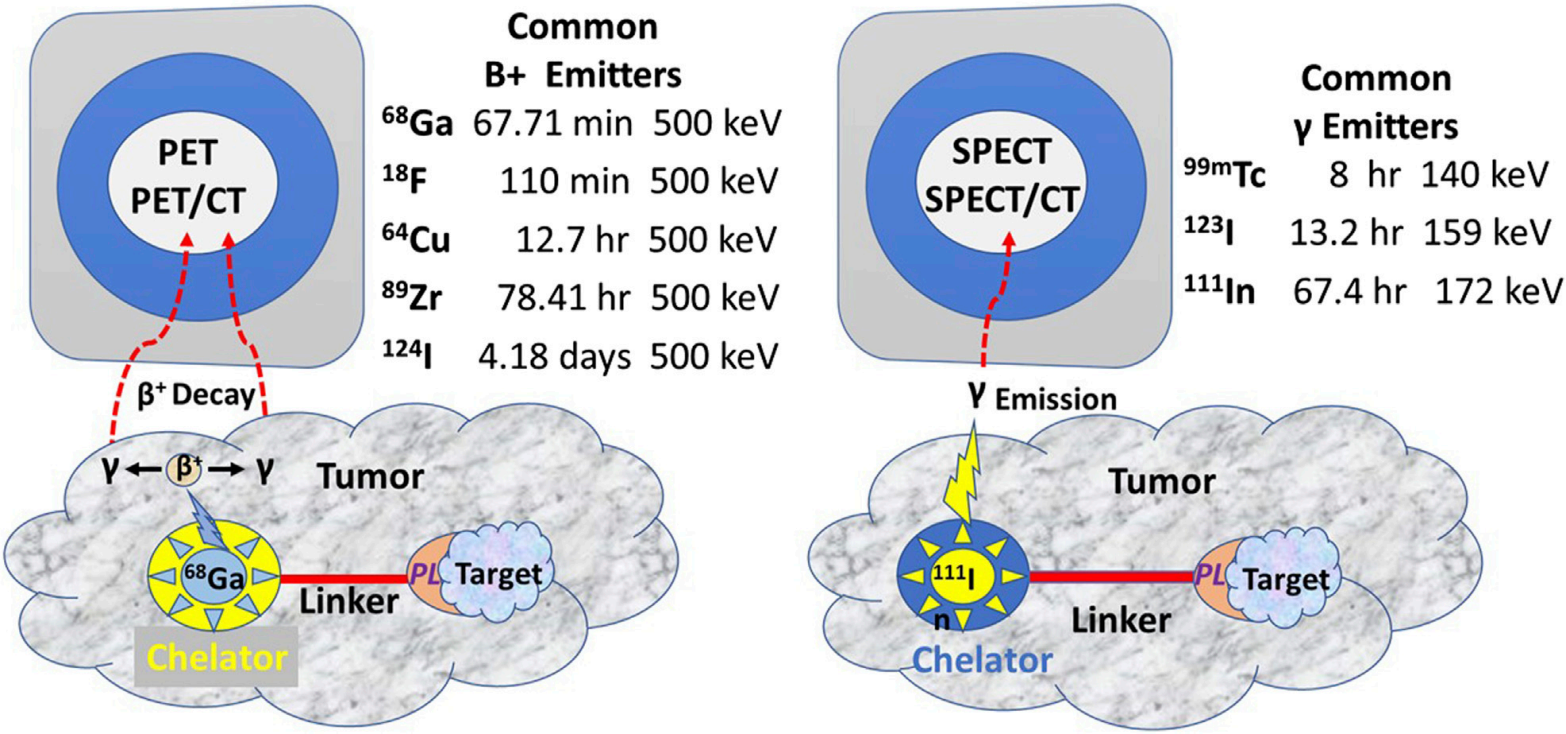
Commonly Used Radionuclides for PET and SPECT Imaging (After [13] PET, Positron emission tomography; SPECT, Single-photon emission computed tomography; CT, Computerized tomography; PL, Preferential locator.
